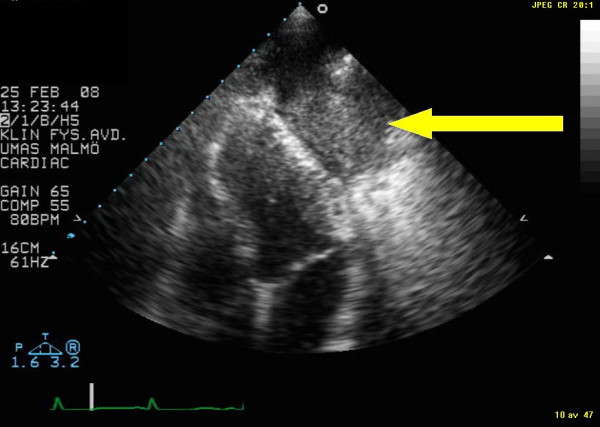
Display of echocardiography image from apical four-chamber view. The arrow indicates the non-small cell lung cancer.
